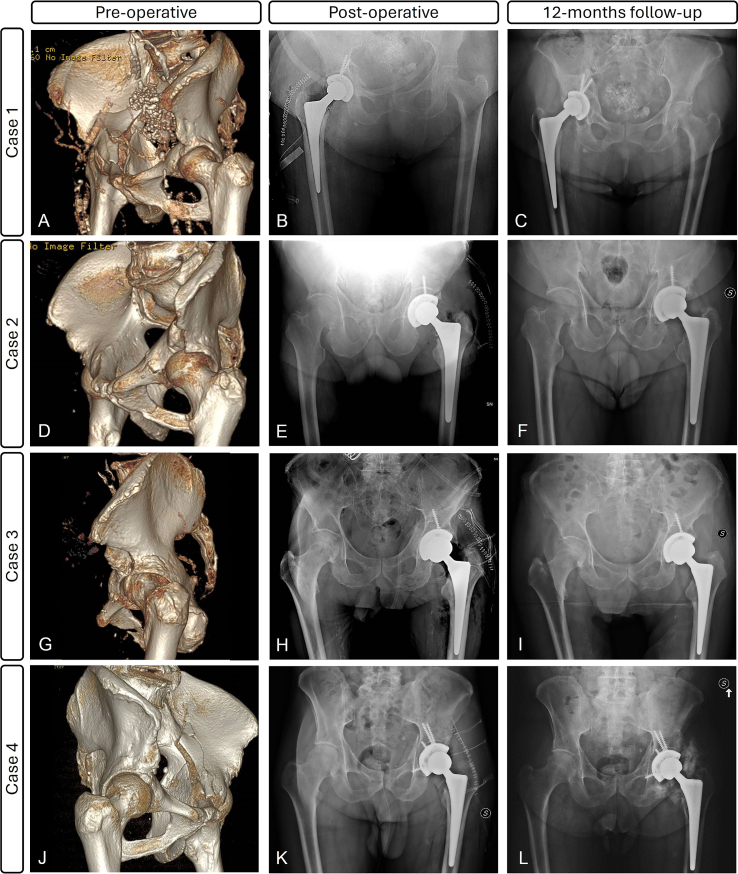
Preoperative 3D reconstruction images, postoperative and follow-up X-rays of the four cases. Case 1 received 2 screws into the ilium to fix the anterior wall fracture. For case 2 and case 3, one screw was deemed sufficient to increase the cup fixation and secure bone fragments. Case 4 had both columns fractured fixed with four screws. Brooker III heterotopic ossification was clearly visible at final follow-up.
